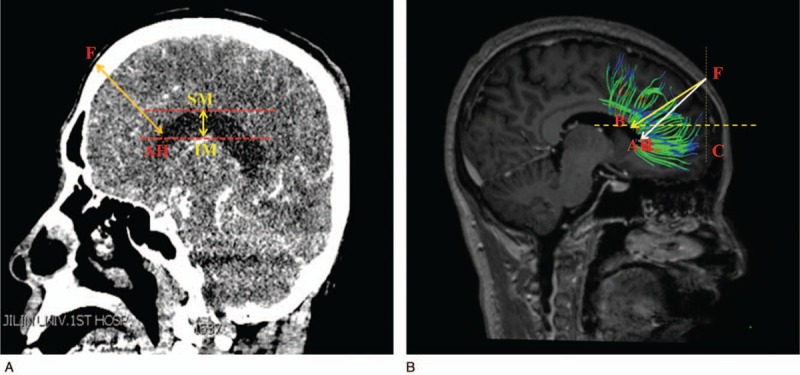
(A) The data and the puncture point from frontal lobe in computed tomography image. (B) The puncture point and angle in diffusion tensor image.
